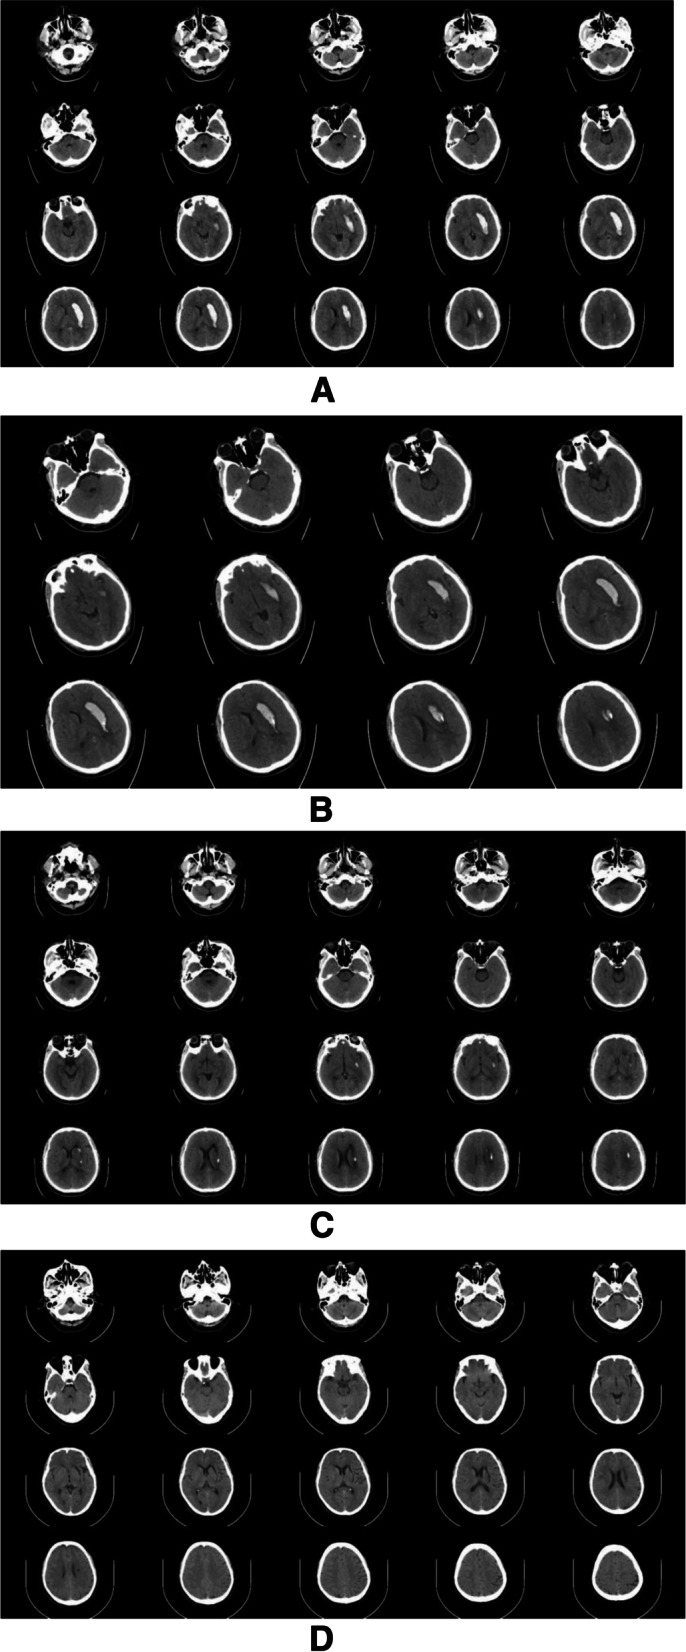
CT of patients with cerebral haemorrhage in the basal ganglia undergoing stereotactic catheterization and drainage. A. Preoperative head CT showed that the amount of bleeding was approximately 25 ml. B. The deformed position of three-dimensional CT on the first day after the operation indicates that the drainage tube is in a good position. C. On the third day after the operation, CT of the head showed that the haematoma was almost completely drained. D. On the 30th day after the operation, CT showed the soft focus after haematoma drainage
